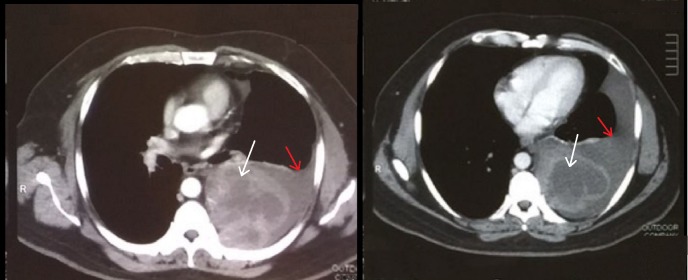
Preoperative axial CT scan of the thorax A large, left-sided posterior mediastinal mass is seen paravertebrally (white arrows) and is associated with a large, left-sided pleural effusion (red arrows). CT= computed tomography
